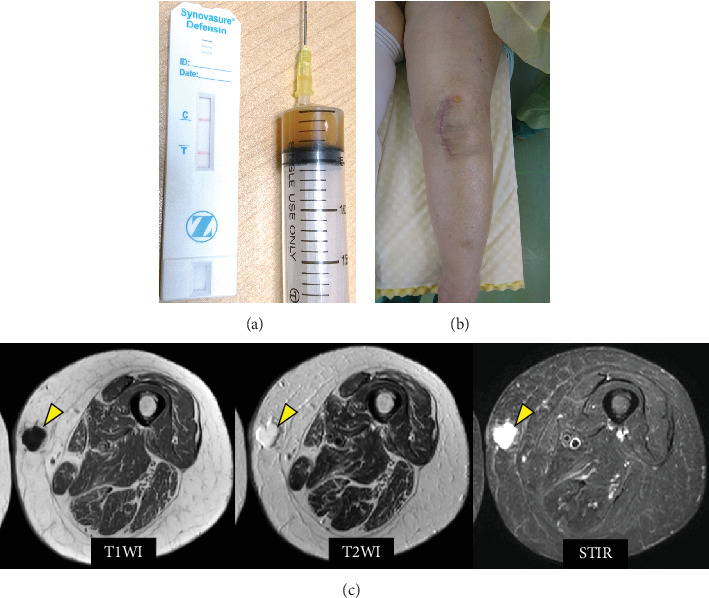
(a) Synovial fluid of the left knee showing yellow and mildly turbid fluid. The alpha-defensin test from this sample revealed positive lines. (b) The affected limb just prior to DAIR showing lower limb edema. (c) MRI of the thigh after the infection subsided. The cyst showing hypointense in T1WI, isointense in T2WI, and hyperintense in STIR. The subcutaneous cyst had shrunk (yellow arrow).
